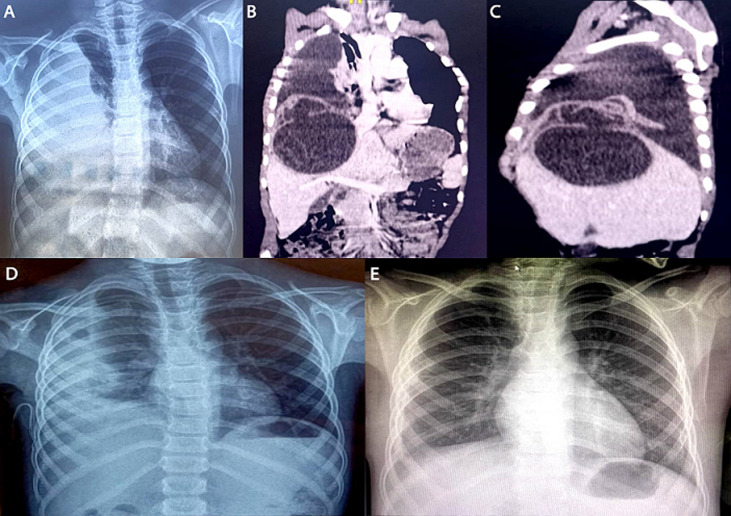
A) chest X-ray film on admission showing right-sided huge pleural effusion with no mass effect; B) coronal computed tomography scan showing huge liver hydatid cyst and a huge pleural effusion; C) sagittal computed tomography scan showing a large liver hydatid cyst communicating with the pleural cavity through diaphragmatic hole; D) chest X-ray film 2 weeks post-operative showing an encysted pleural effusion; E) chest X-ray film 3 months later showing disappearance of the pleural fluid
